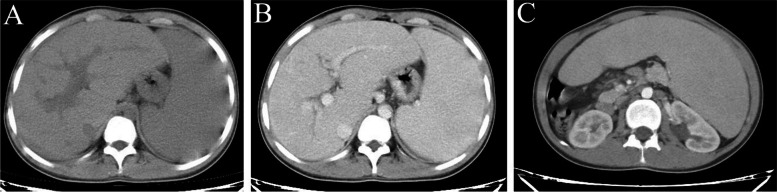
The results of CT scan. A Plane CT scan; B Delayed phase of contrast-enhanced CT; C CT scan shows giant spleen and normal kidneys
